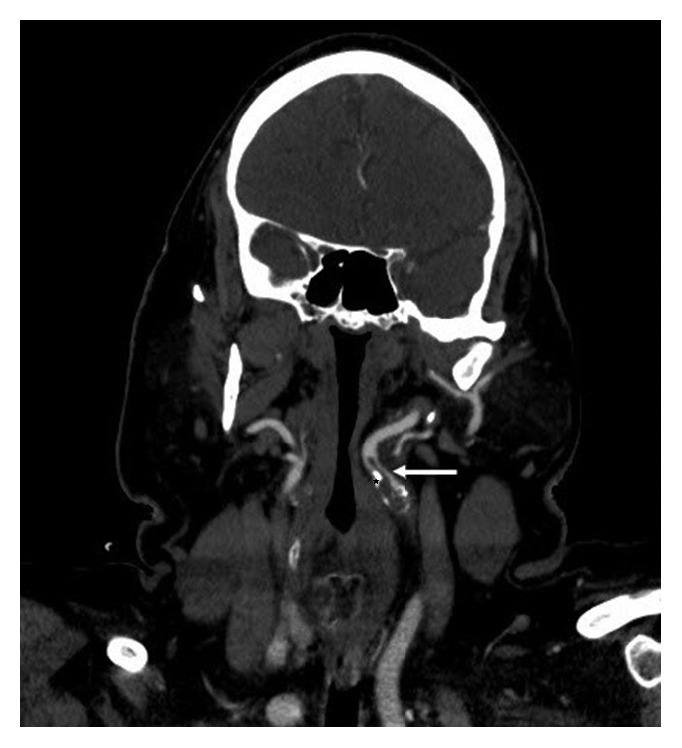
Coronal reformatted view from CT angiogram shows an intraluminal filling defect within the proximal left internal carotid artery consistent with thrombus (white arrow). There is also adjacent calcified atheromatous plaque (black asterisk). The internal carotid arteries are tortuous and deviate medially into the retropharyngeal space.
